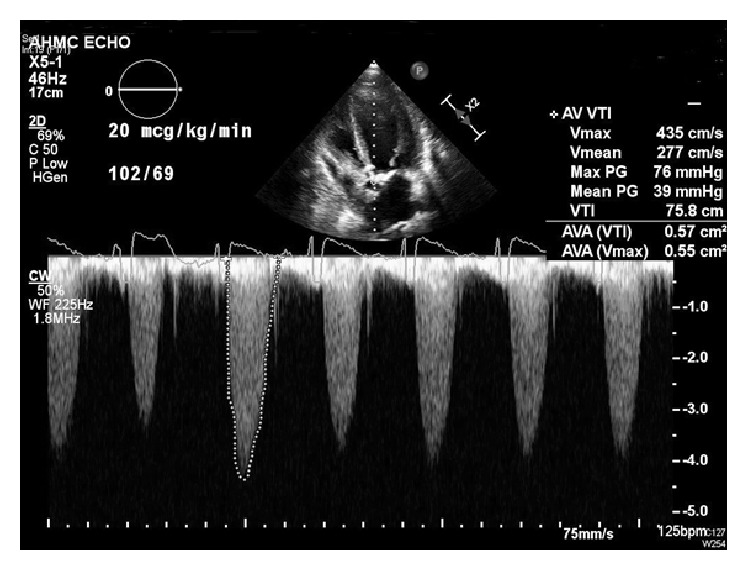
Dobutamine stress echocardiogram (DSE) at 20 mcg/kg/min of dobutamine. AVA: aortic valve area, HGen: midrange frequency, PG: pressure gradient, Vmax maximum velocity, Vmean: mean velocity, and VTI: velocity time integral.
